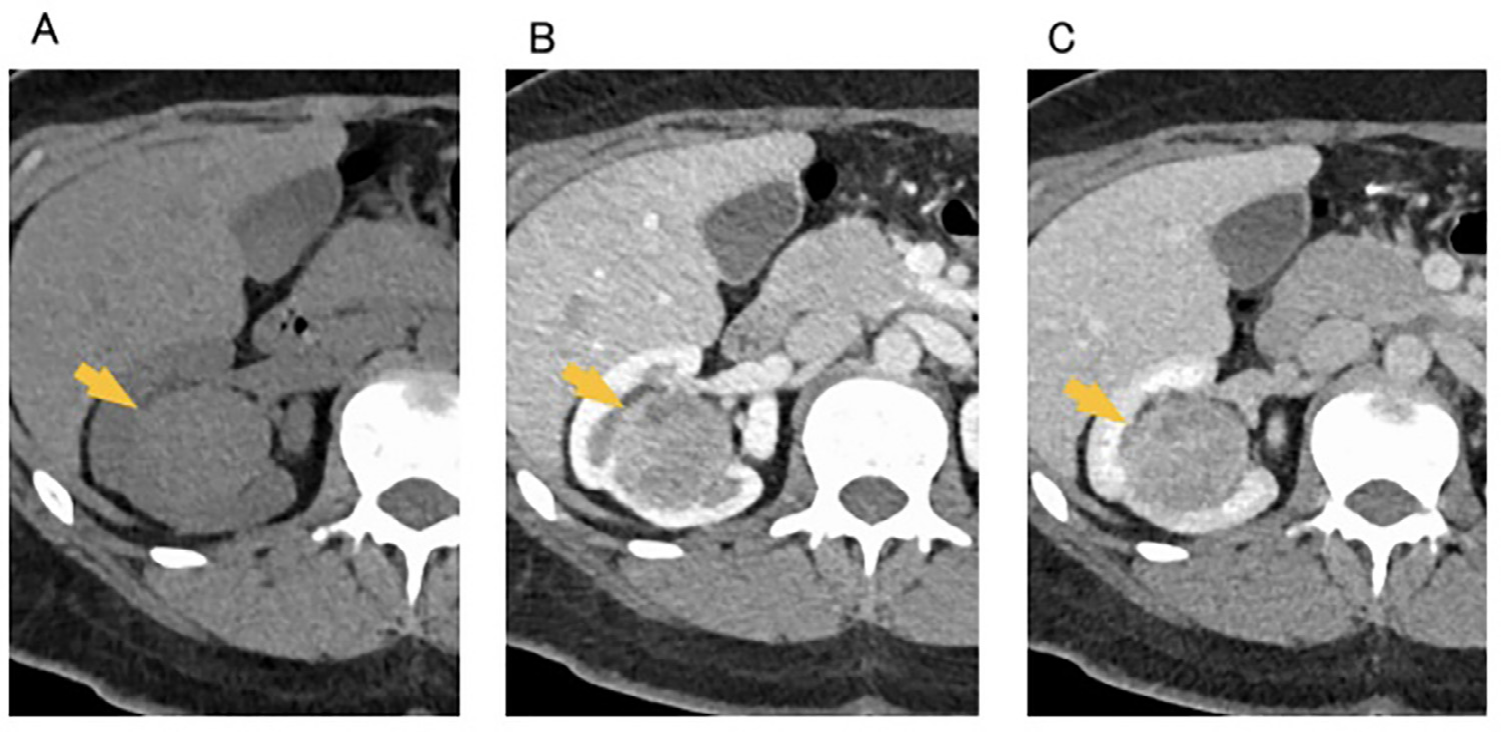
Dynamic contrast-enhanced CT. (A) unenhanced, (B) corticomedullary phase, and (C) nephrogenic phase. There is a 4 cm × 3.5 cm × 4 cm tumor with smooth margins and well-defined borders in the right renal sinus (yellow arrow). On unenhanced CT, the tumor appears to have a homogeneous density of 46 HU (A). Dynamic contrast-enhanced imaging demonstrates relatively uniform and gradual enhancement, with 73 HU in the corticomedullary phase and 104 HU in the nephrogenic phase (B, C).
